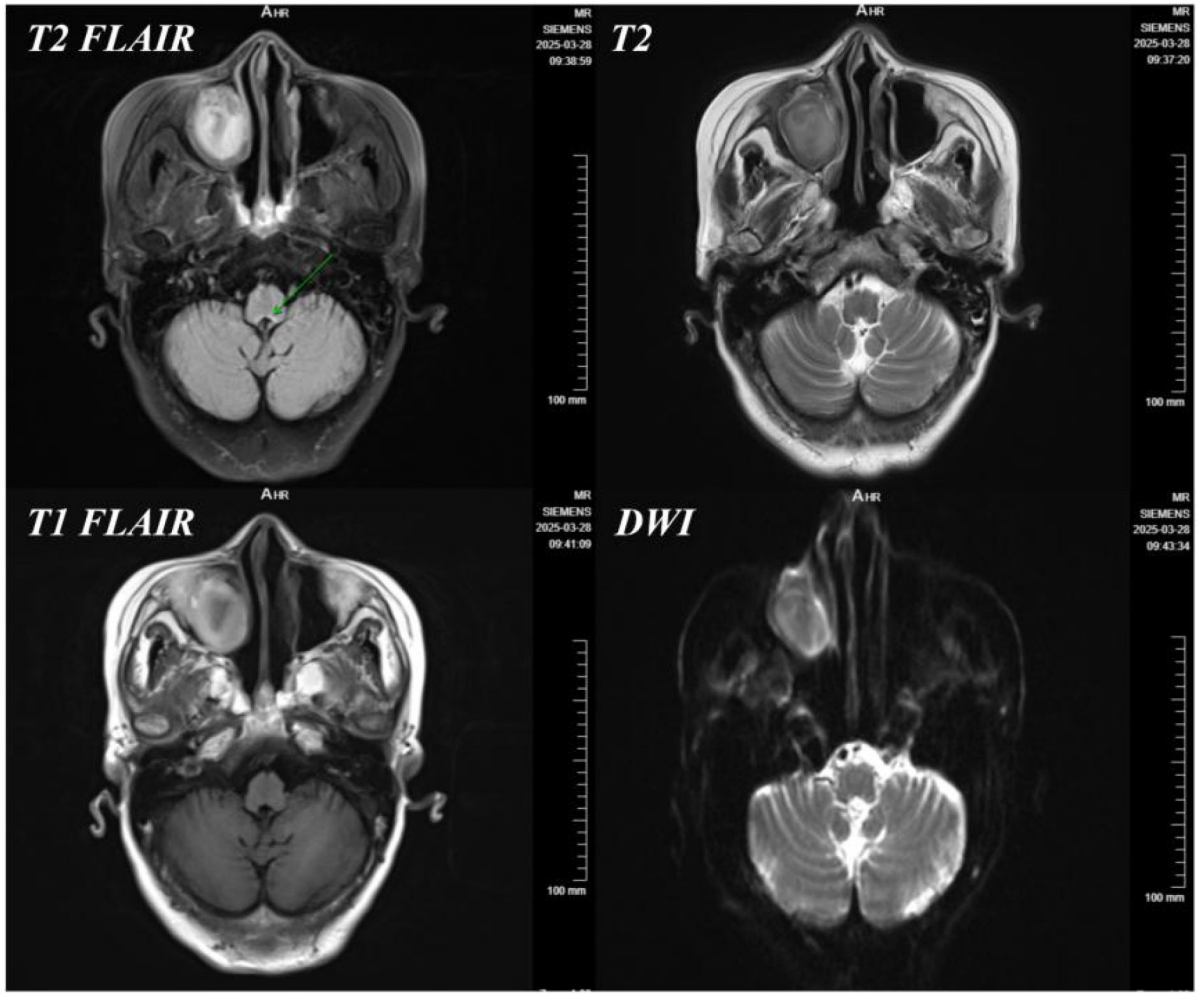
Cranial MRI of the patient on March 28, 2025.
